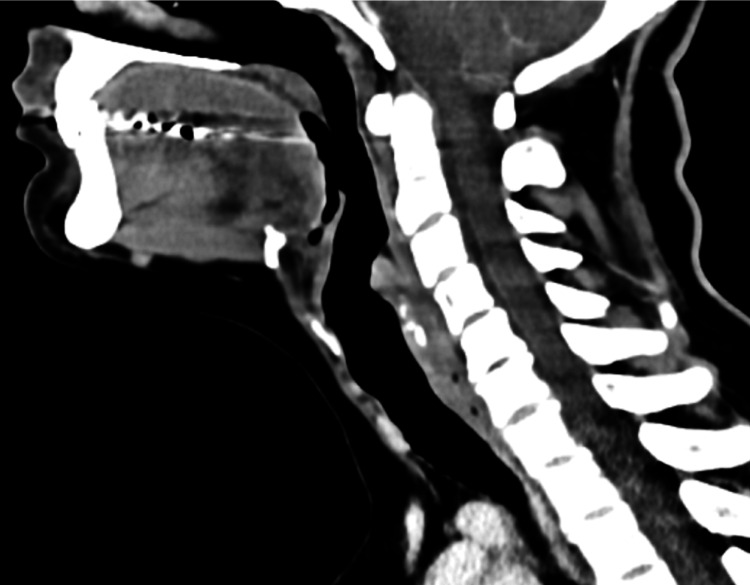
Cervical computed tomography with no clear evidence of pharyngeal pouch.
